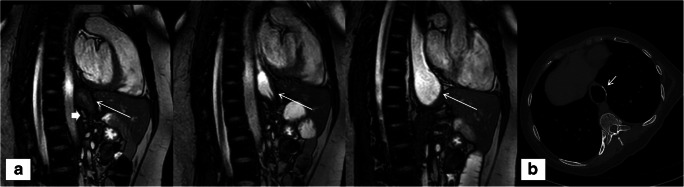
A 50-year-old female who underwent RYGB, pouch resizing, and banding (a short, bold arrow); dynamic MRI shows filling of the pouch during fluid intake (a long arrows). Moderate ITM was suspected and confirmed by CT (b short arrow indicates staple lines above the diaphragm)
